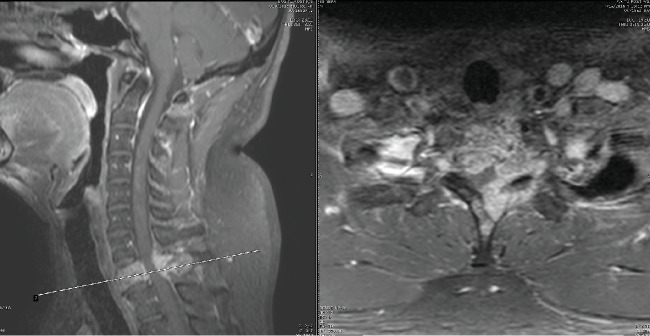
Preoperative T1 magnetic resonance imaging showing sagittal and axial views.
